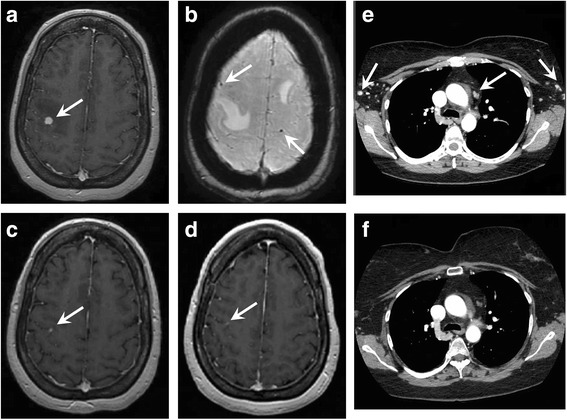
Axial post-contrast FSPGR brain MRI was performed 3 months after the initiation of ipi/nivo in the setting of new neurologic symptoms, revealing 6 new enhancing parenchymal lesions, the largest in the right parietal lobe (a, arrow). Multiple additional foci of chronic hemorrhage without associated visible enhancement are demonstrated on susceptibility-weighted imaging (b, arrow). Concurrent thoracic CT revealed interval decrease in size and contrast enhancement of several axillary and mediastinal lymph nodes (compare arrows in e with f). Post-contrast MRI performed one month later showed marked decrease in the size of the right parietal lesion (c, arrow), and resolution of all of the previously enhancing lesions. The patient was tapered off steroids without significant change in imaging after four months (d, arrow)
